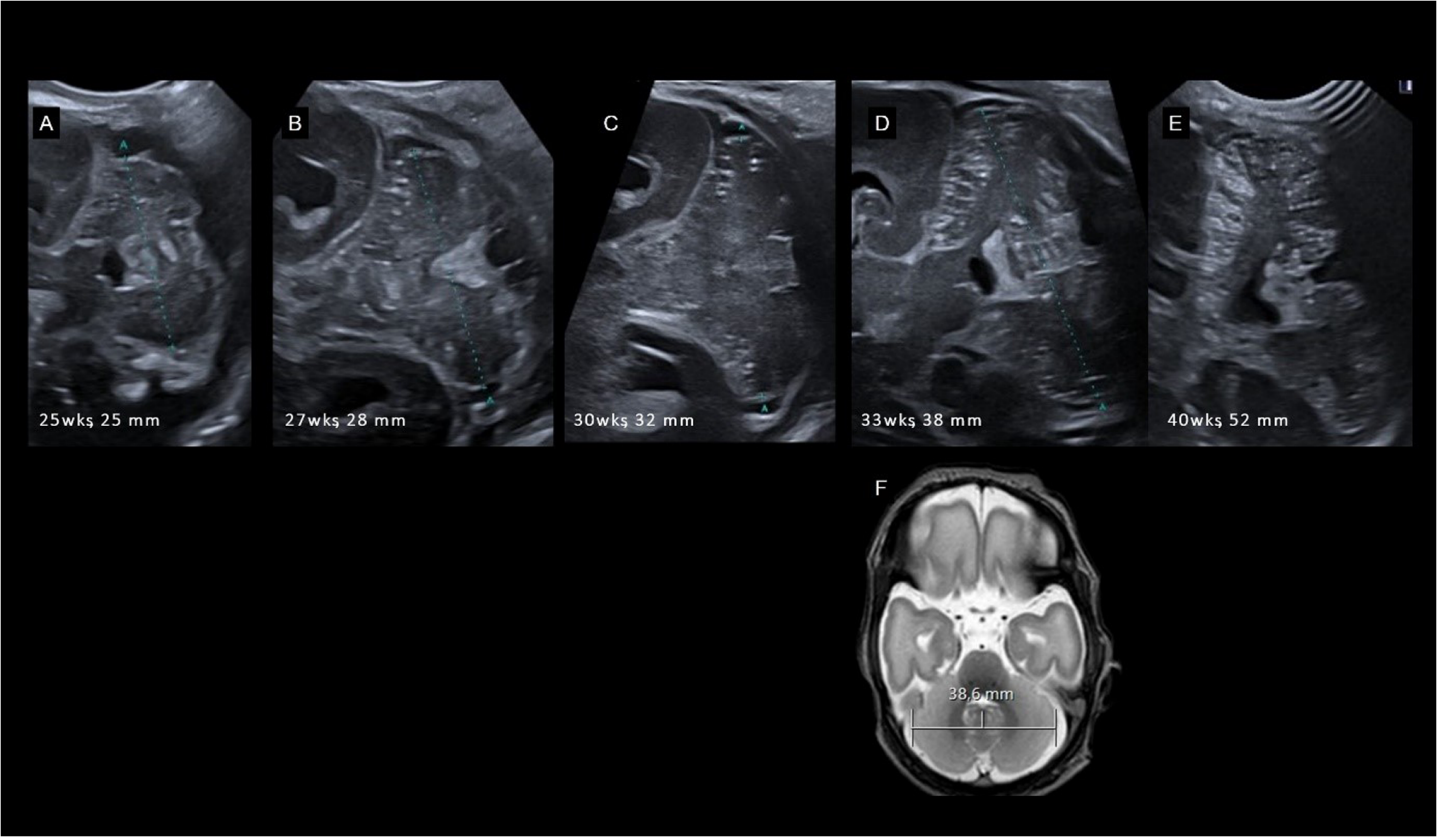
Unimpaired cerebellar growth in a preterm infant of 25 weeks gestational age. Serial coronal ultrasound scans (A-E) through the mastoid fontanel show a normal development with proceeding foliation representing the rapid increase in cerebellar surface. (F) T2 MRI scan at postmenstrual age of 33 weeks results in same transverse cerebellar diameter compared to corresponding ultrasound scan (D)
